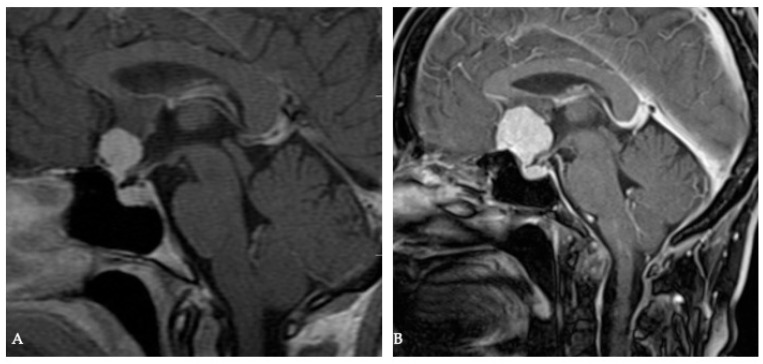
Meningiomas develop along the path of least resistance. A 53-year-old female presented in 2013 with an incidental finding of meningioma of the tuberculum sellae (A). She refused surgical treatment. In 2017 she presented with visual disturbance in the right eye. The MRI outlined the growth of the tumor (B). Meningiomas of the tuberculum sellae are restrained by the arachnoid covering the ICA and posterior communicating arteries, optic nerves, optic chiasm, lamina terminalis, pituitary stalk, infundibulum, and Lilequist membrane. Consequently, the only route for tumor spread is anteriorly over the planum, over the optic nerves, and above the chiasm around the anterior cerebral artery complex. The growth over the planum may be the result of an anatomic defect in the arachnoid of the chiasmatic cistern at the chiasmatic sulcus or a postfixed chiasm.
